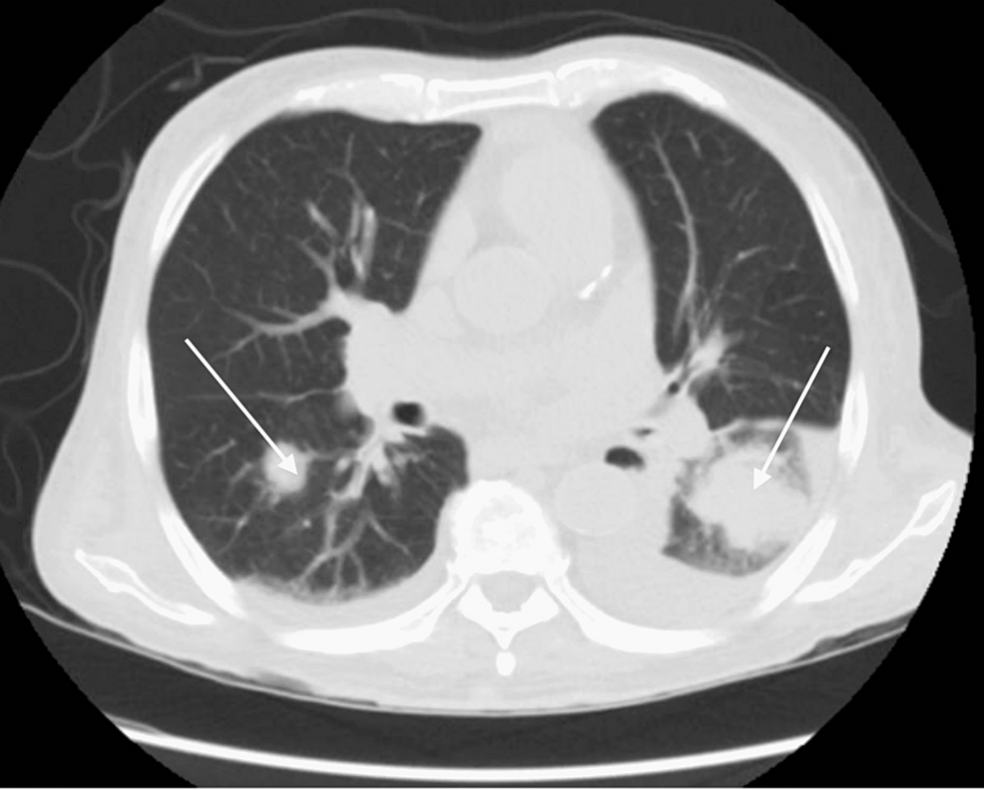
White arrows show left lower lobe infiltrate and right lung nodule on chest CT. CT: computed tomography
